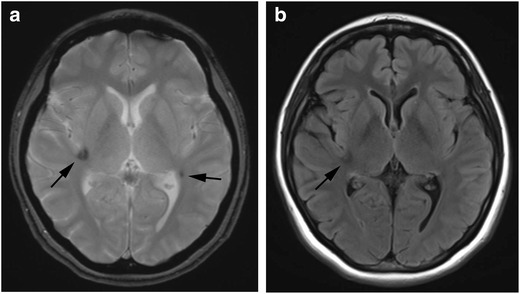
Radiation induced focal haemosiderin deposition. A 29-year-old woman, who received radiation therapy for acute lymphocytic leukaemia, had no symptom. a A T2*-weighted image shows focal haemosiderin deposition in the right insular and left temporo-parietal lobe (*arrows*). b FLAIR image shows slight low intensity area in the right insular (*arrowheads*). Left temporo-parietal small lesion is not apparent (*arrow*)
